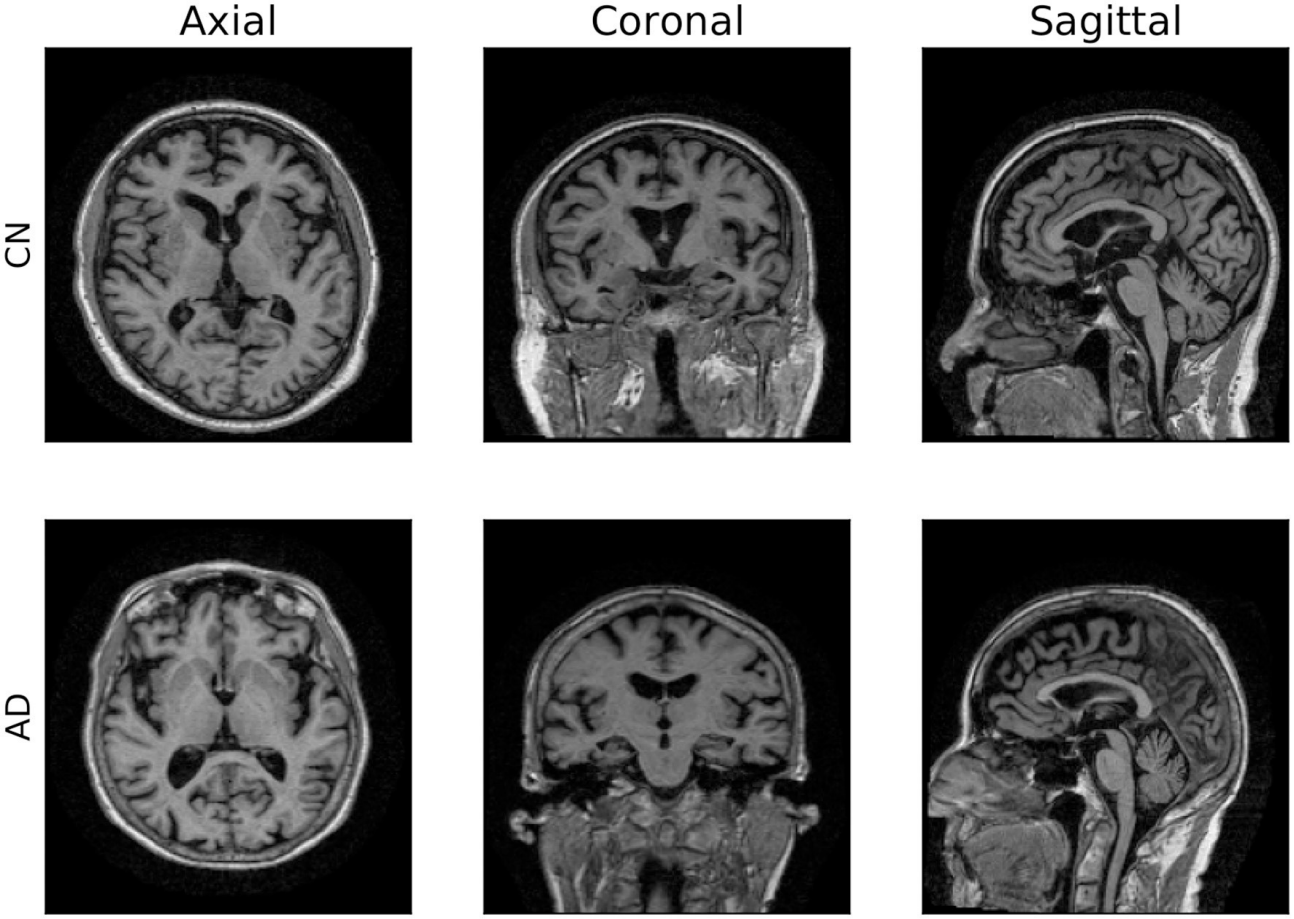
2D visualization of 3D MRI scans. Axial, coronal and sagittal planes of two brain images from ADNI dataset.
